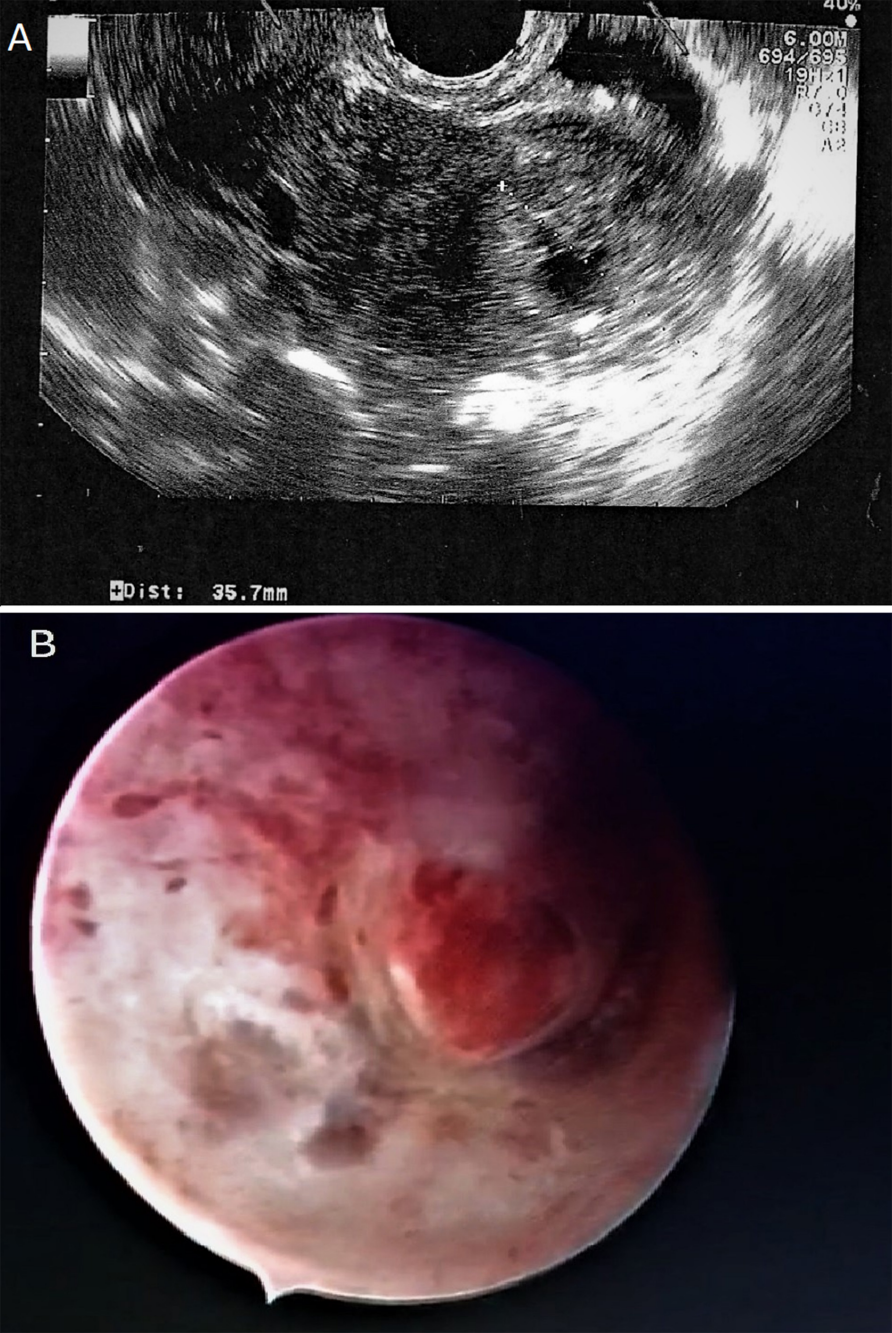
Interstitial pregnancy. (A) Coronal view of the uterus on transvaginal ultrasound showing an empty cavity with a mass of 35.7 mm in diameter. (B) Hysteroscopy shows the ectopic interstitial pregnancy localized in the left tubaric corner.
